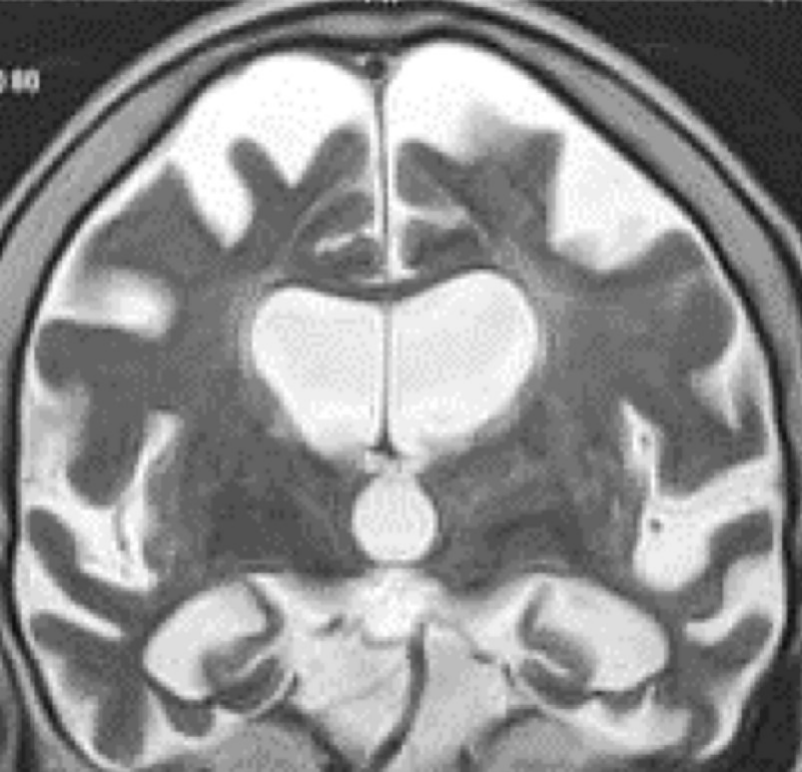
Coronal magnetic resonance imaging demonstrated significant asymmetric diffuse cortical atrophy, especially in the frontotemporal lobes, being slightly more to the left.
